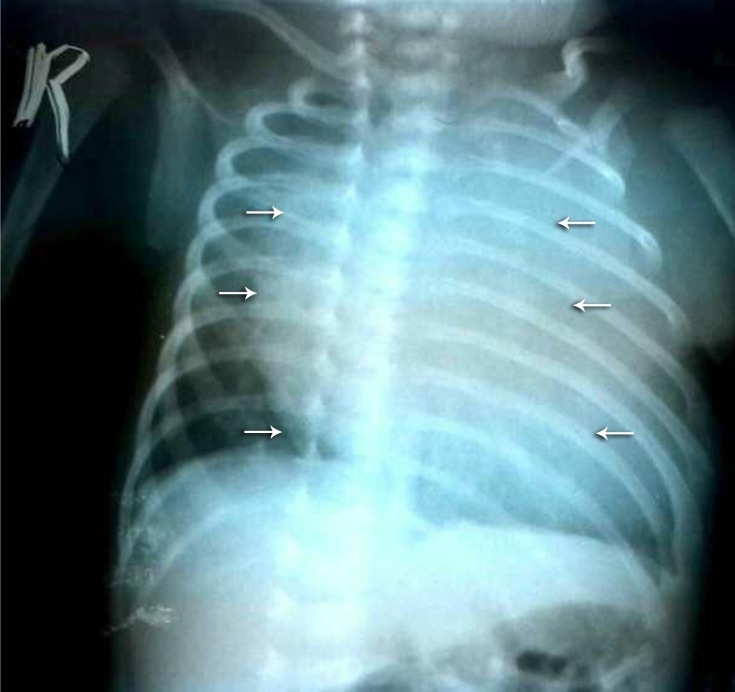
Chest radiograph )anteroposterior view), revealing cardiomegaly and evidence of a pericardial mass. The arrows show the border of the possible mass.
